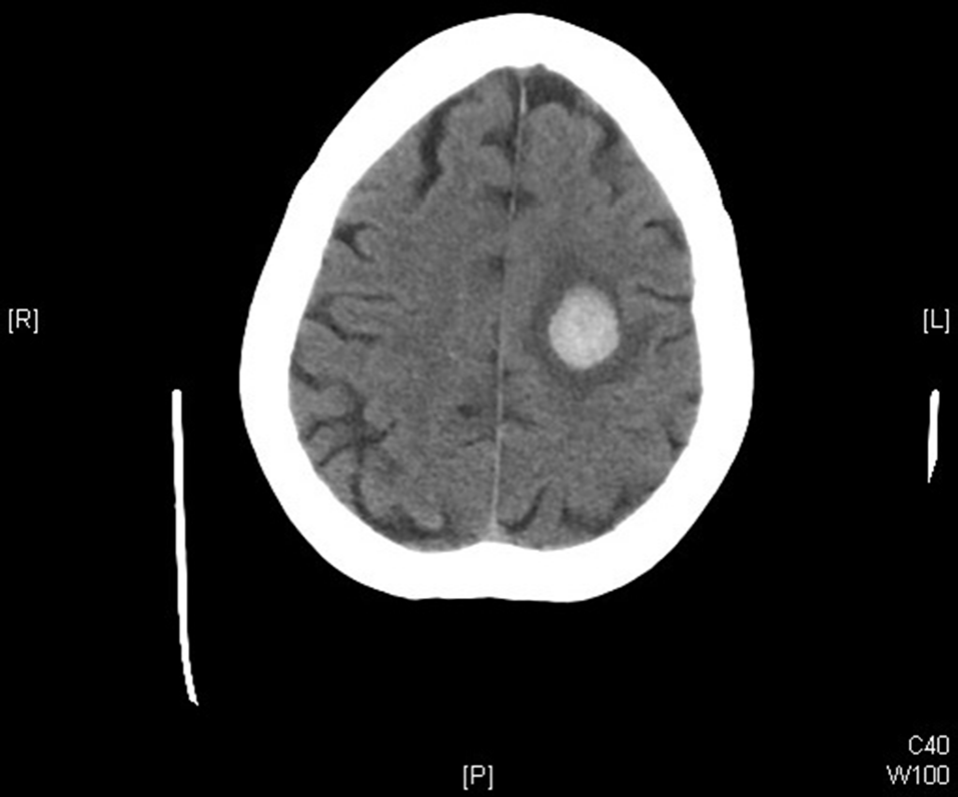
Non-contrast CT demonstrating left parietal lobe hemorrhage
